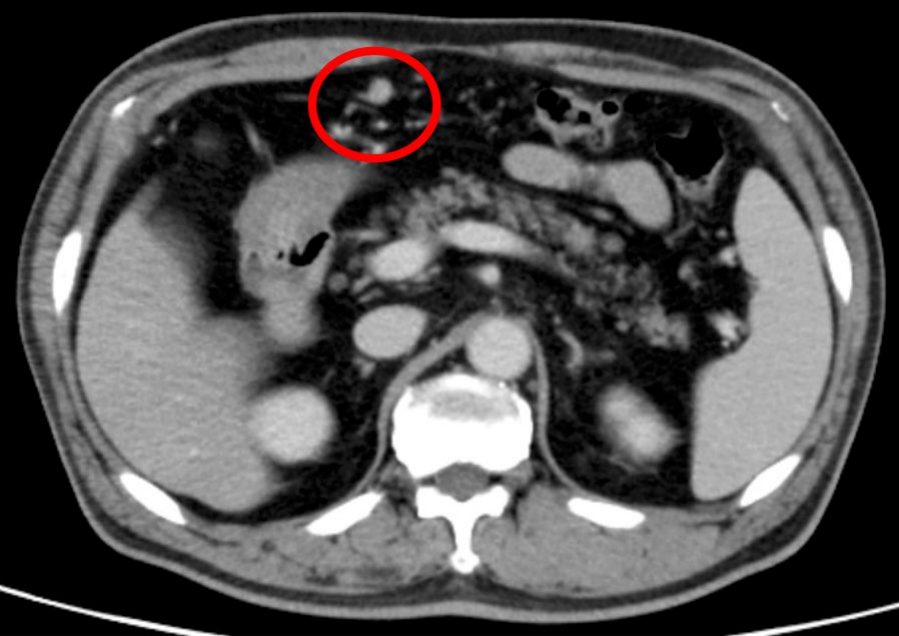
Computed tomography (CT) findings at 32 years of follow-up. CT revealed regional lymphadenopathy with a 10-mm diameter in the greater omentum along the gastroepiploic artery
